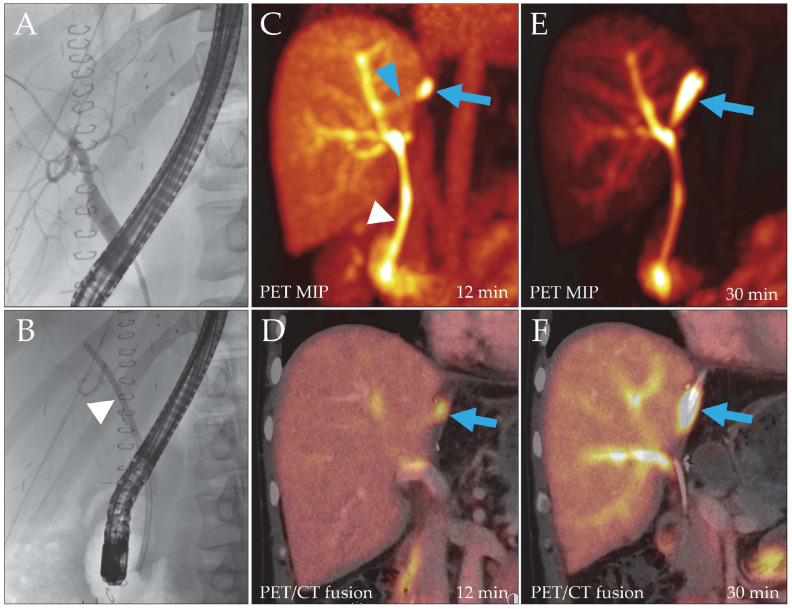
Patient 2, liver recipient. Post-transplantation ERC (A) did not reveal a bile leak, but to facilitate biliary drainage, a hepatoenteric stent was placed ((B), arrowhead). Due to persistent drainage of bile through the catheter, a DAZA-PET/CT was performed. Despite a patent stent ((C), white arrowhead), it showed a leakage site at the liver resection surface from 12 min after tracer injection ((C,D), blue arrow; SUVmax 29.7/SUVmax/mean liver tissue 9.9/7.8) draining into a bilioma, which filled over time ((E,F), blue arrows). A small bile duct branch appeared to reach the leak from the main right hepatic ducts ((C), blue arrowhead). MIP, maximum intensity projection.
